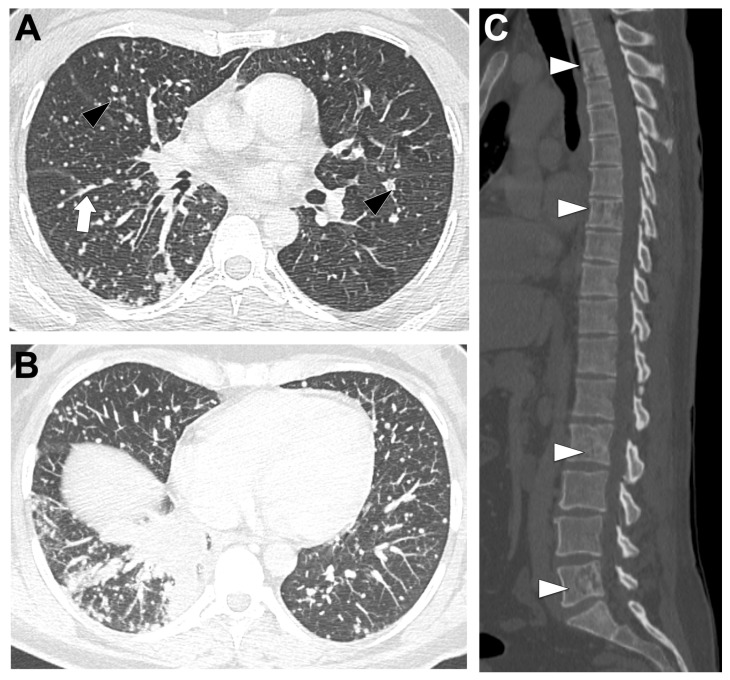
Representative baseline CT imaging findings in patients with HER2-altered lung adenocarcinoma (LUAD). A 50-year-old never-smoking woman presented with multiple pulmonary nodules (black arrowhead) and a pleural nodule along the fissures (white arrow) (A,B). Bone-window CT revealed multiple sclerotic and lytic vertebral metastases (white arrowheads) (C). Molecular analysis identified an in-frame exon 20 HER2 substitution.
